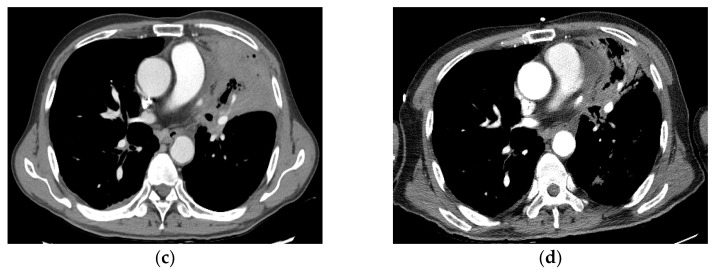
Case 3 (patient no. 19). (a) A 68-year-old man with obstructive pneumonitis had a left upper lobe mass obstructing the left upper lobe segmental bronchus. He also had pseudoaneurysm of the pulmonary artery. He was recommended to undergo pneumonectomy. He underwent SBRT at 55 Gy in 10 fractions because of underlying pneumoconiosis and COPD. (b) Follow-up imaging at 2 months showing PR after SBRT. (c) Chest CT 4 months after SBRT showing tumor necrosis with progression of radiation pneumonitis/tracheobronchitis or combined infection, with diffuse wall thickening of the trachea and left main bronchus, accompanied by pericardial effusion. (d) Follow-up imaging 6 months after SBRT showing improved pneumonitis and aggravation of the dilated pulmonary artery inside the consolidation in the left upper lobe. However, massive hemoptysis was observed 6.3 months after SBRT, which resulted in death. CT, computed tomography; SBRT, stereotactic body radiotherapy; PR, partial response; COPD, chronic obstructive pulmonary disease.
